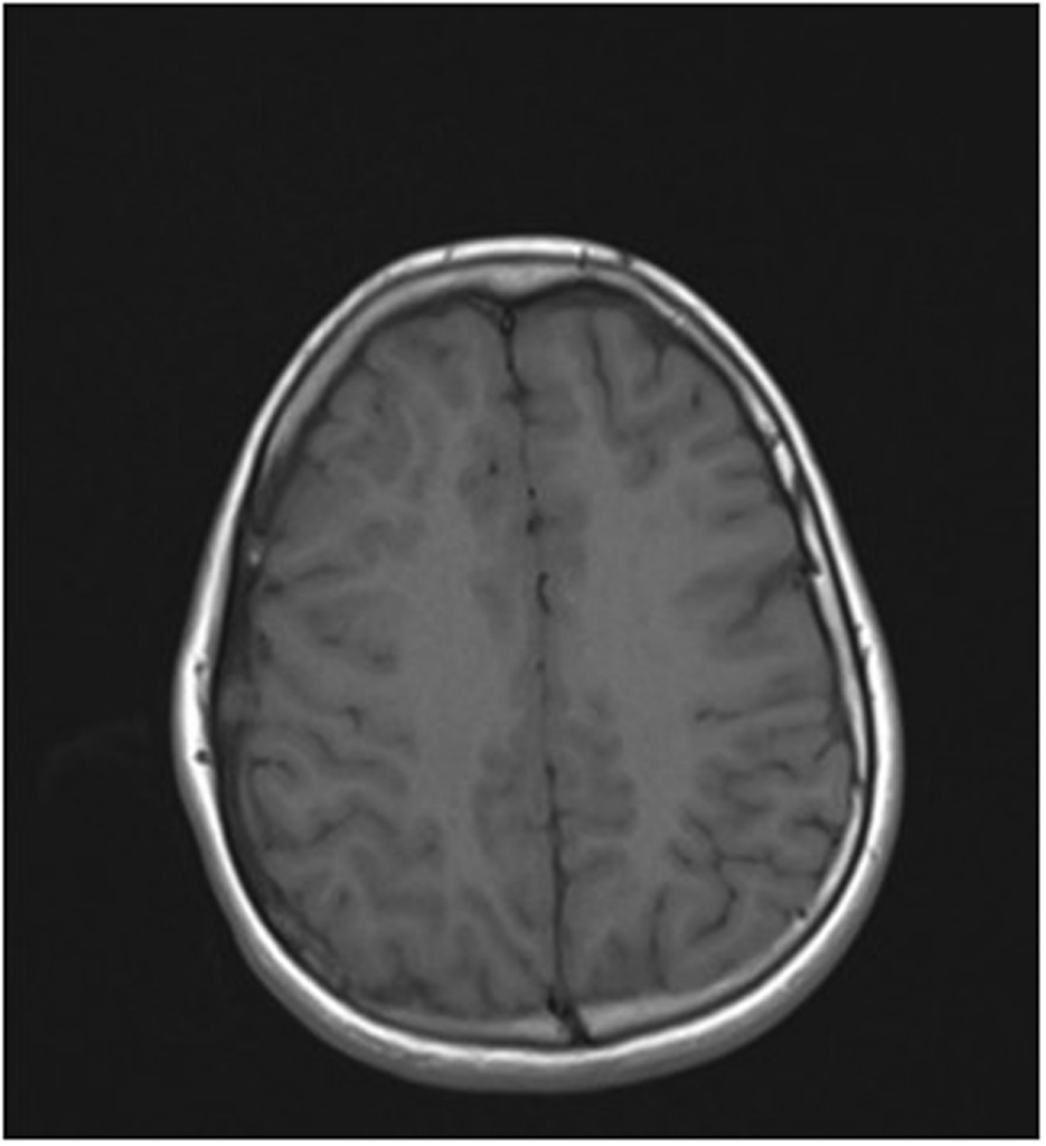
Axial T1‐weighted MR image shows an isointense lesion in the diploic space. Diploic space widening is depicted
